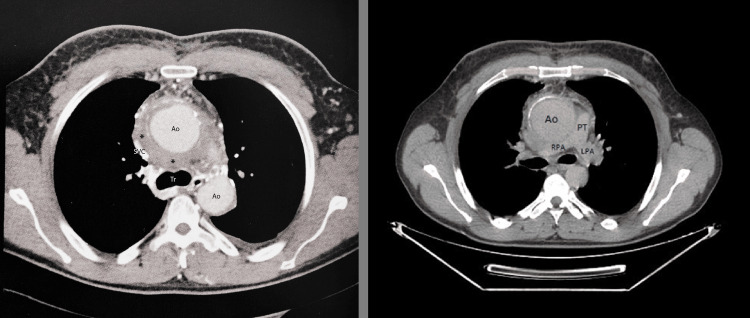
Angiogram (left) and non-contrast CT (right) showing aneurysmal dilation of the ascending aorta with periaortic infiltration (*) causing lamination of the SVC Ao: Thoracic Aorta; SVC: Superior Vena Cava; Tr: Tracheal Carina; PT: Pulmonary trunk; RPA: Right Pulmonary Artery; LPA: Left Pulmonary Artery
